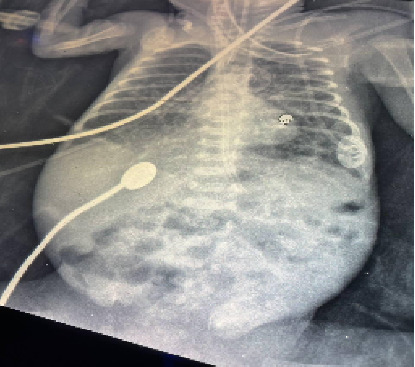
The x-ray shows pneumatosis intestinalis with multiple small air bubbles along the bowel wall, suggestive of necrotizing enterocolitis.
